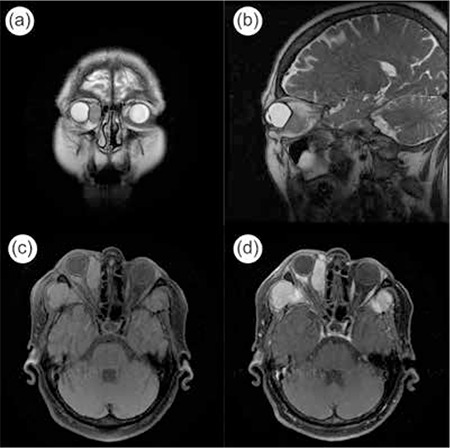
The mass located in the orbit visualized in three different planes by magnetic resonance imaging (a, b and c), and by contrast-enhanced magnetic resonance imaging (d)
